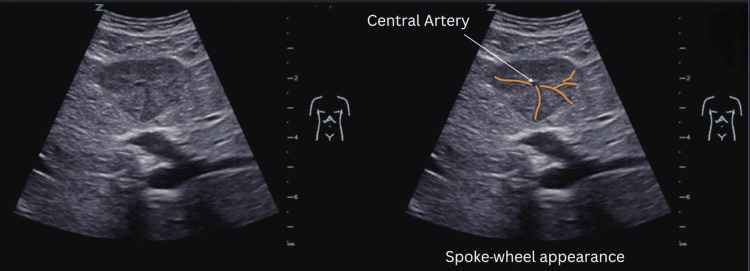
Native ultrasound showing spoke-wheel pattern with centrifugal radiating vessels originating from the central artery typical of FNH FNH: focal nodular hyperplasia
